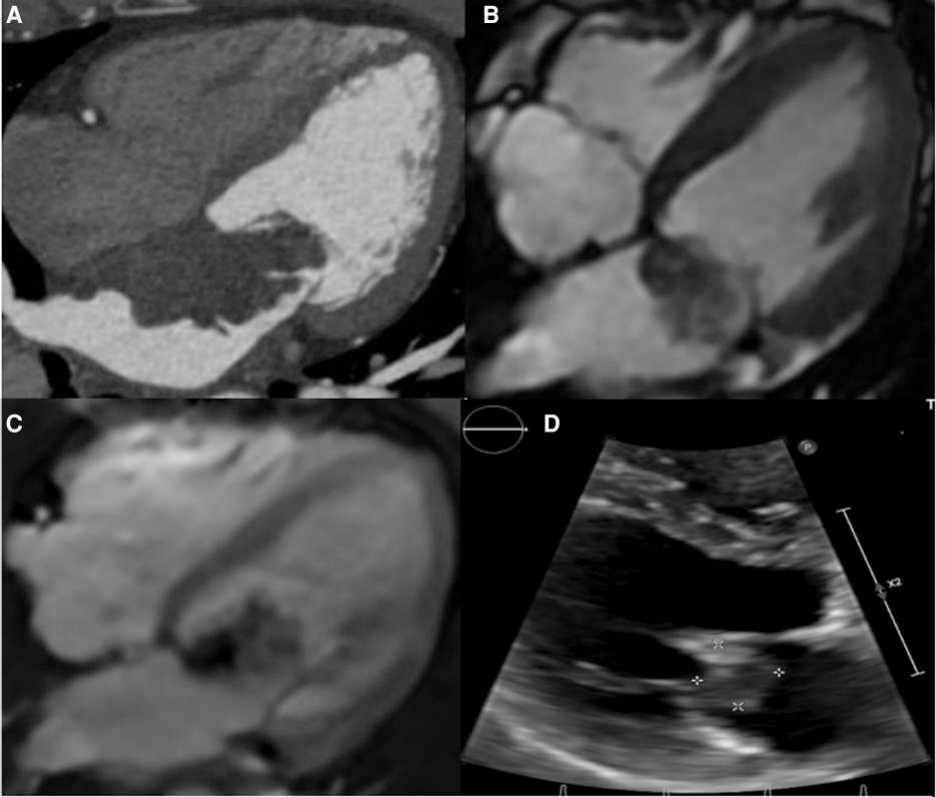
Tumour recurrence on cardiac CT, MRI, and echocardiogram. *(A*) CT image demonstrates a large polypoid left atrial mass along the interatrial septum obstructing the mitral inflow. *(B*) HLA FIESTA image demonstrates a polypoid left atrial mass obstructing the mitral inflow. *(C*) Phase-sensitive HLA LGE image demonstrates the presence of LGE in a polypoid left atrial mass consistent with malignancy. This mass is a biopsy-proven sarcoma. *(D*) Follow-up echocardiogram 2 months after revealed a 1.7 cm × 1.5 cm mass on the anterior leaflet of the mitral valve.
